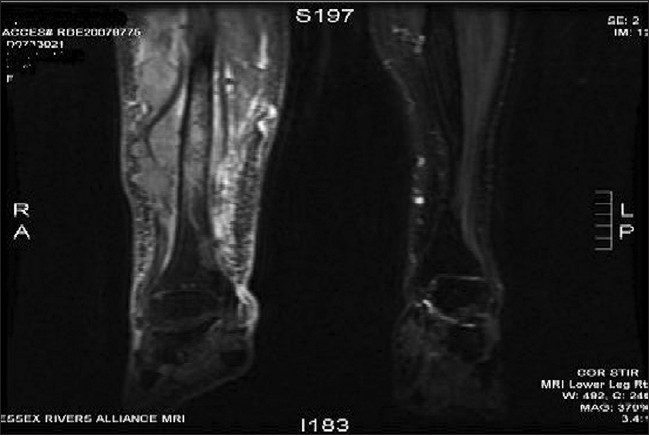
MRI of right leg showing malignant infiltration of tibia in its mid and lower third with adjacent soft tissue masses
